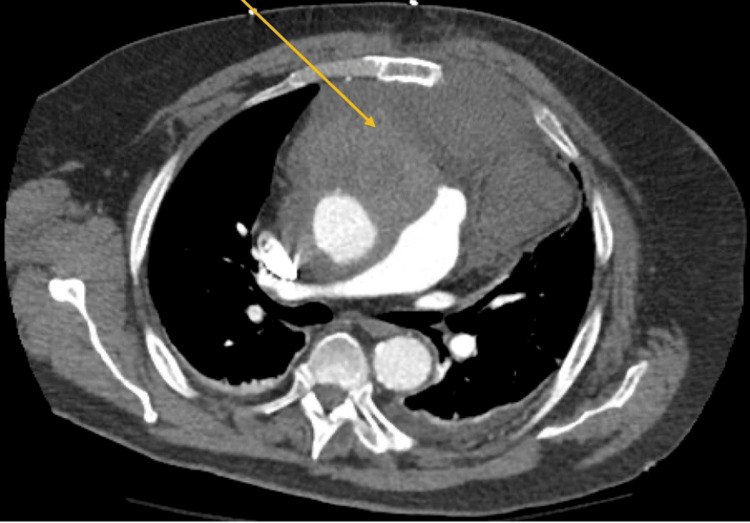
Significant expansion of the mediastinal mass on repeat CT.
